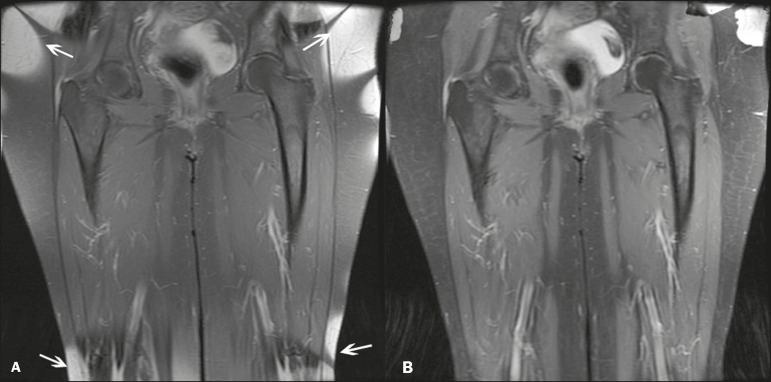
Contrast-enhanced coronal images of the thighs of a 59-year-old female patient with various pulse sequences: T1-weighted FSE sequence with fat suppression (A) and T1-weighted IDEAL sequence (B). Clear presence of artifacts at the edges of image A due to heterogeneity of the B0 or B1 magnetic field when using a wide field of view, impairing fat suppression.
